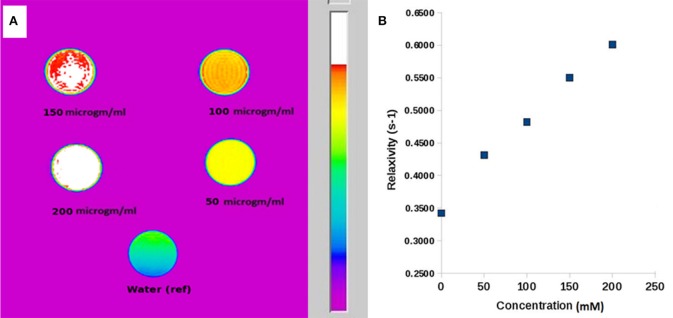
(A) T1-weighted MR image of ICDHA substituted samples dispersed at different concentrations in distilled water and (B) longitudinal relaxivity vs. concentration graph of ICDHA.
